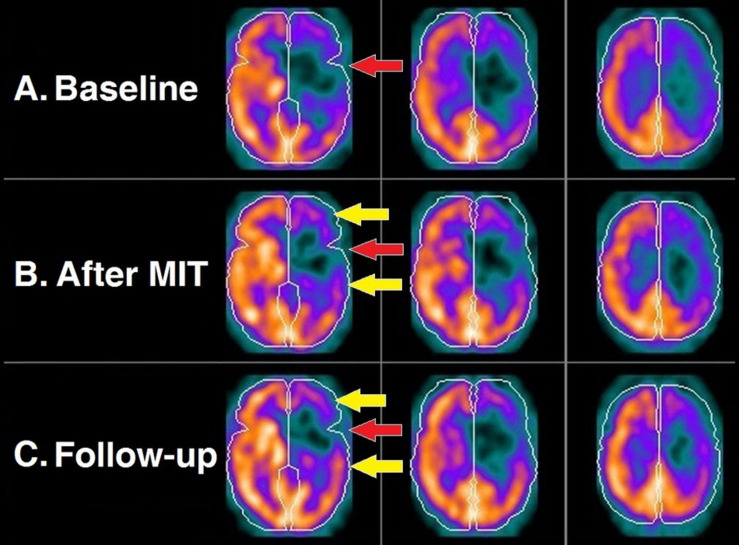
Brain perfusion scintigraphy at the three time points of the study: before MIT (A); right after its completion (B); and 3 months later (C). Each row depicts three consecutive transversal SPECT slices of the brain. Perceptible radiotracer uptake (warm colors) represents cortical areas with normal blood supply, as opposed to those exhibiting diminished to negligible uptake (cold colors) denoting mildly to severely compromised perfusion. Expectedly, the necrotic core of the stroke (*red arrow*) remains unaltered in all three scans. However, there is an evident gradual perfusion improvement of the hypoperfused cortex surrounding the necrotic core (*yellow arrows*).
